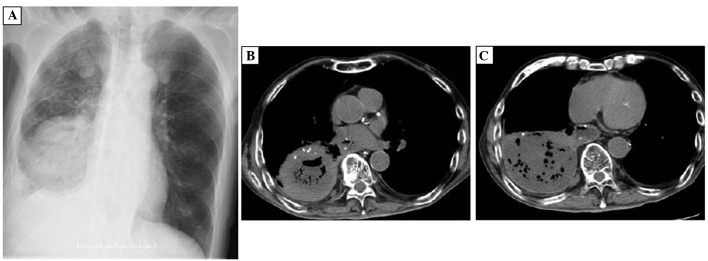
(A) Chest X-ray film on first admission showing a large mass in the right lower lung field. (B and C) Non-enhanced computed tomography scan of the chest on first admission showing a large cavitated mass in the right lower lobe and pleural effusion with multiple gas bubbles.
